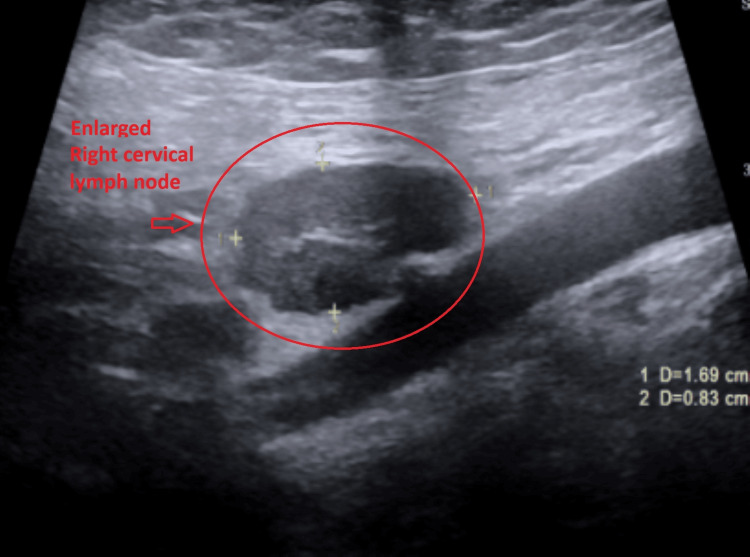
Ultrasound image of the largest right cervical lymph node in the sagittal view
